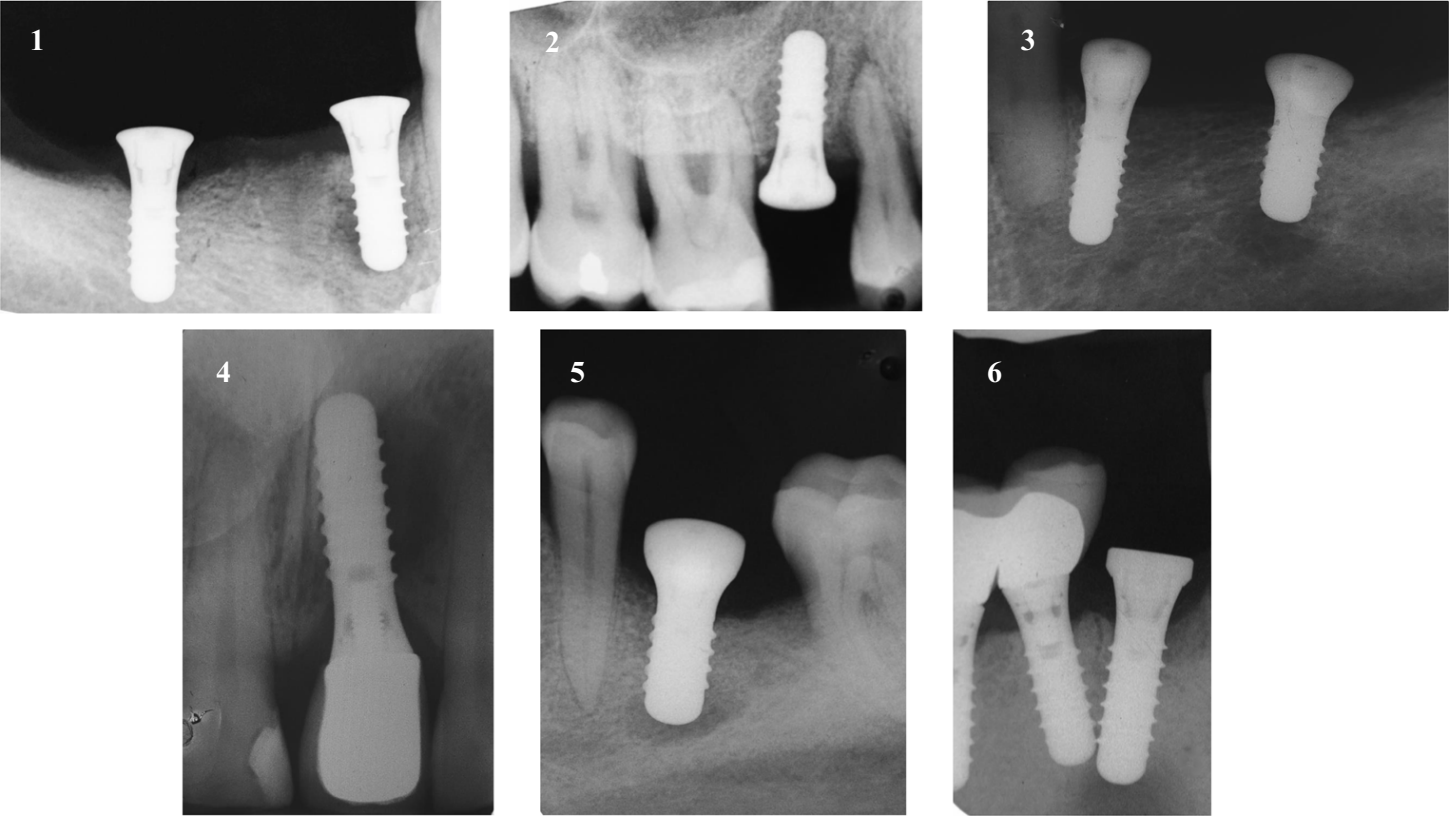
The RPI lesions in the six implants. The first patient described an acute pain in the apical zone of the mesial implant 5 days after implant placement. The second patient reported the same symptoms after 51 days. The third patient presented pain while blowing nose and sneezing in the area of the distal implant after 25 days. The fourth patient came to our observation describing to feel a soft and painful point on the palatal aspect of the implant after 165 days. The fifth patient presented pain, swelling and a fistulous tract over the vestibular aspect of the implant periapical bone after 29 days. The sixth patient showed swelling and redness over the mesial implant area and described pain on percussion 36 days after implant placement
